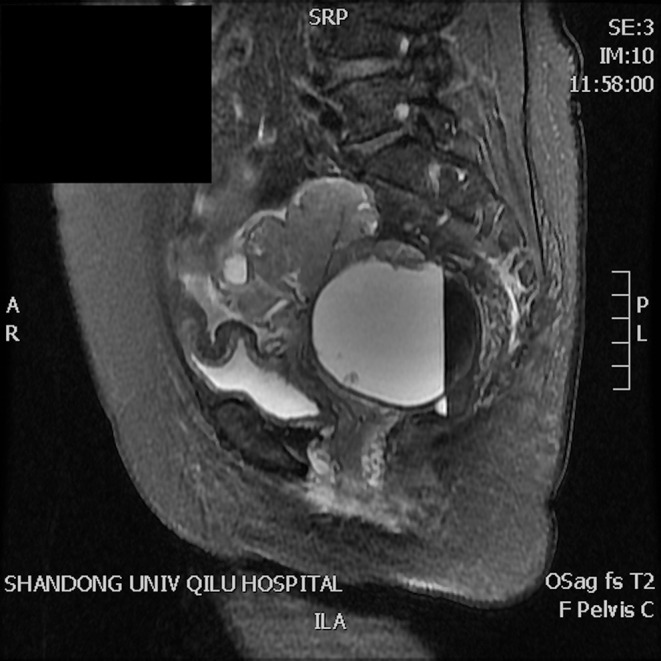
Magnetic resonance imaging revealing solid, cystic, irregular, and space-occupying lesions in the left adnexal area and before the rectum, presenting long T2 signals and equal short T1 signals. The solid part of the diffusion-weighted imaging lesion shows high signal intensity with unclear boundaries, the cystic part shows the liquid level, enhanced scanning of the solid part reveals obvious heterogeneous enhancement, and the boundary between the lesion and adjacent structures is unclear.
